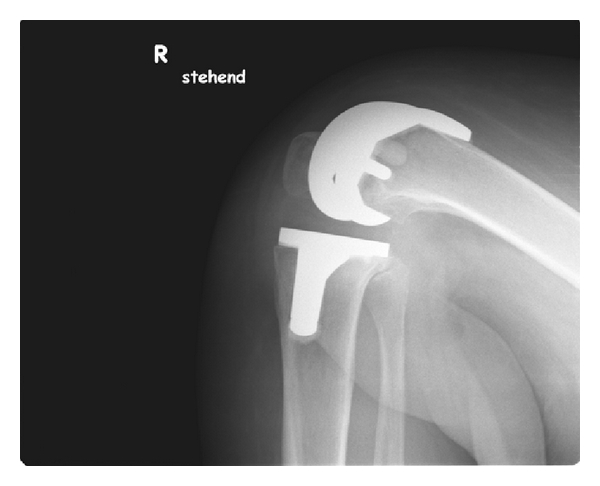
Lateral X-ray of a Columbus knee in maximum flexion: the remaining original posterior condyles do not contact the rim of the polyethylene inlay although there is already full contact of calf and tight. There is no instability or subluxation of the implant.
